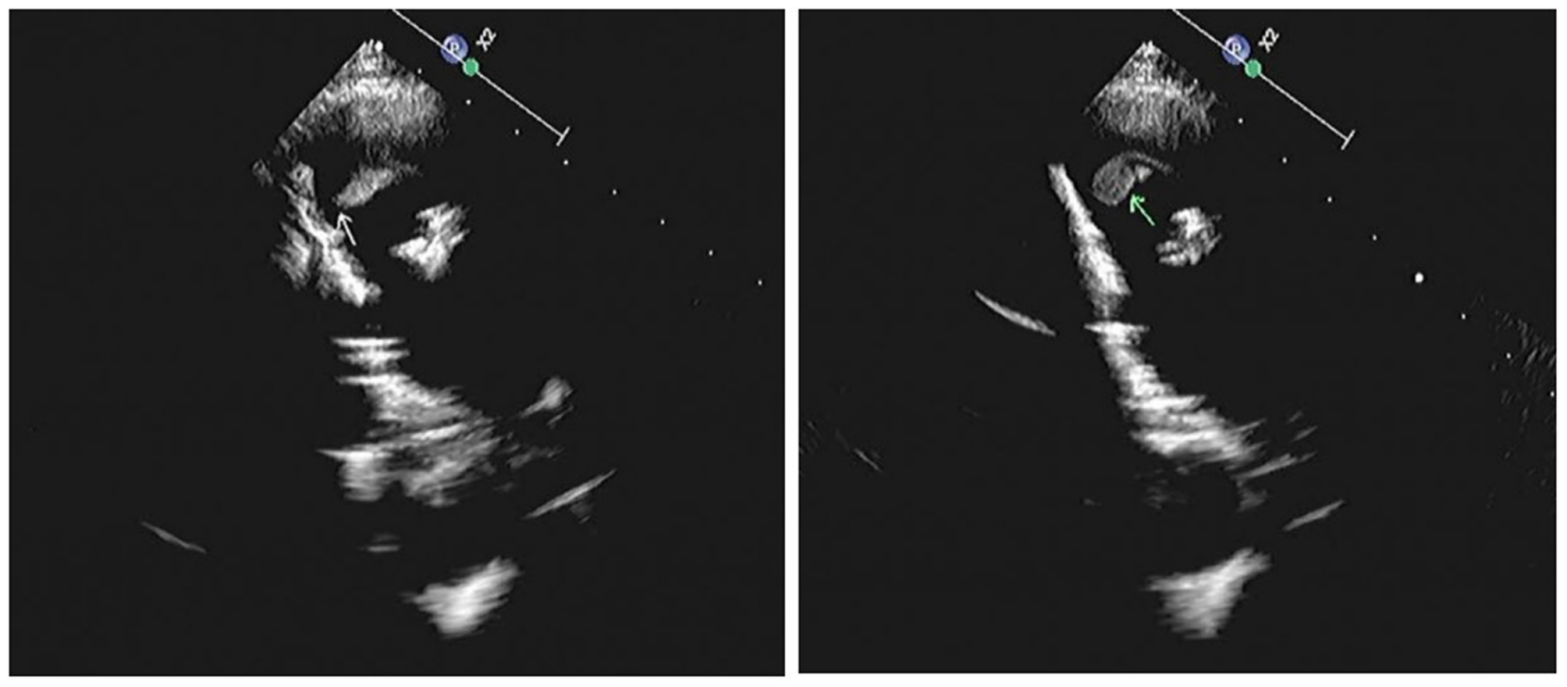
Cardiac ultrasound demonstrated pulmonary valve vegetation (arrow).
